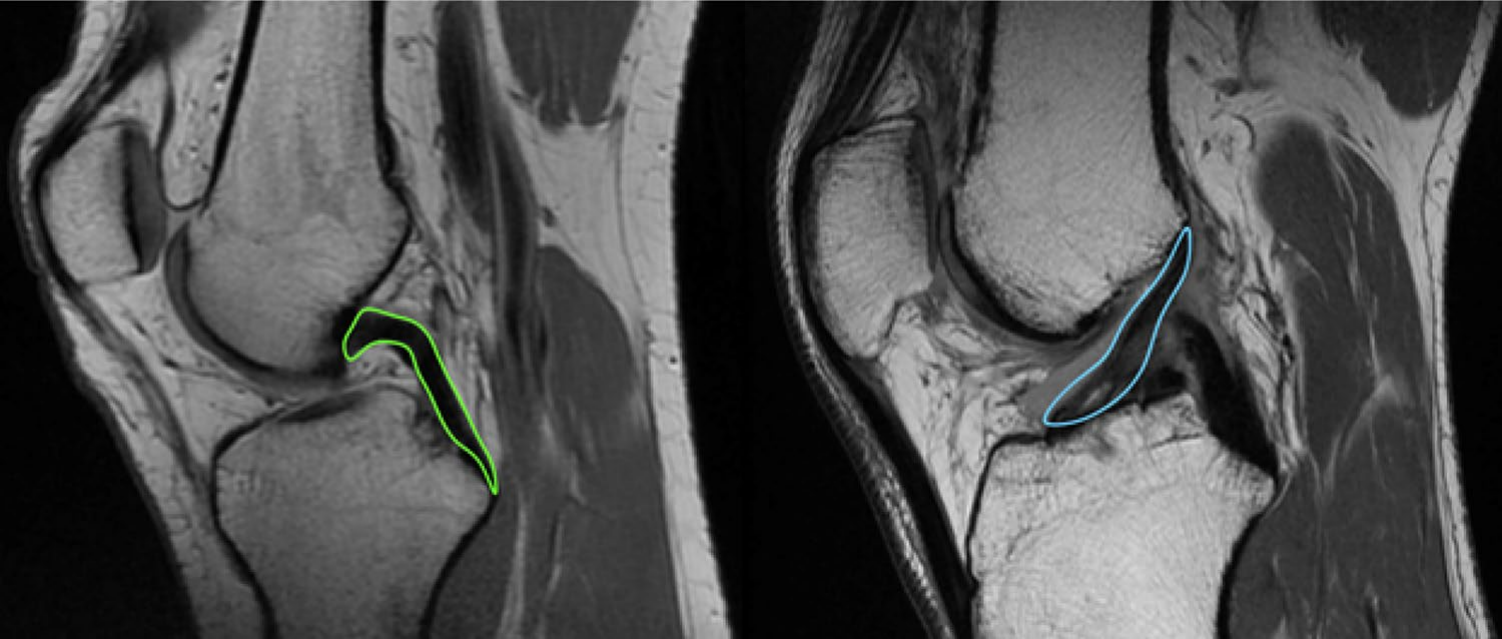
Sagittal view MRI of the knee, in which the PCL (left, green) and the ACL (right, blue) are outlined
